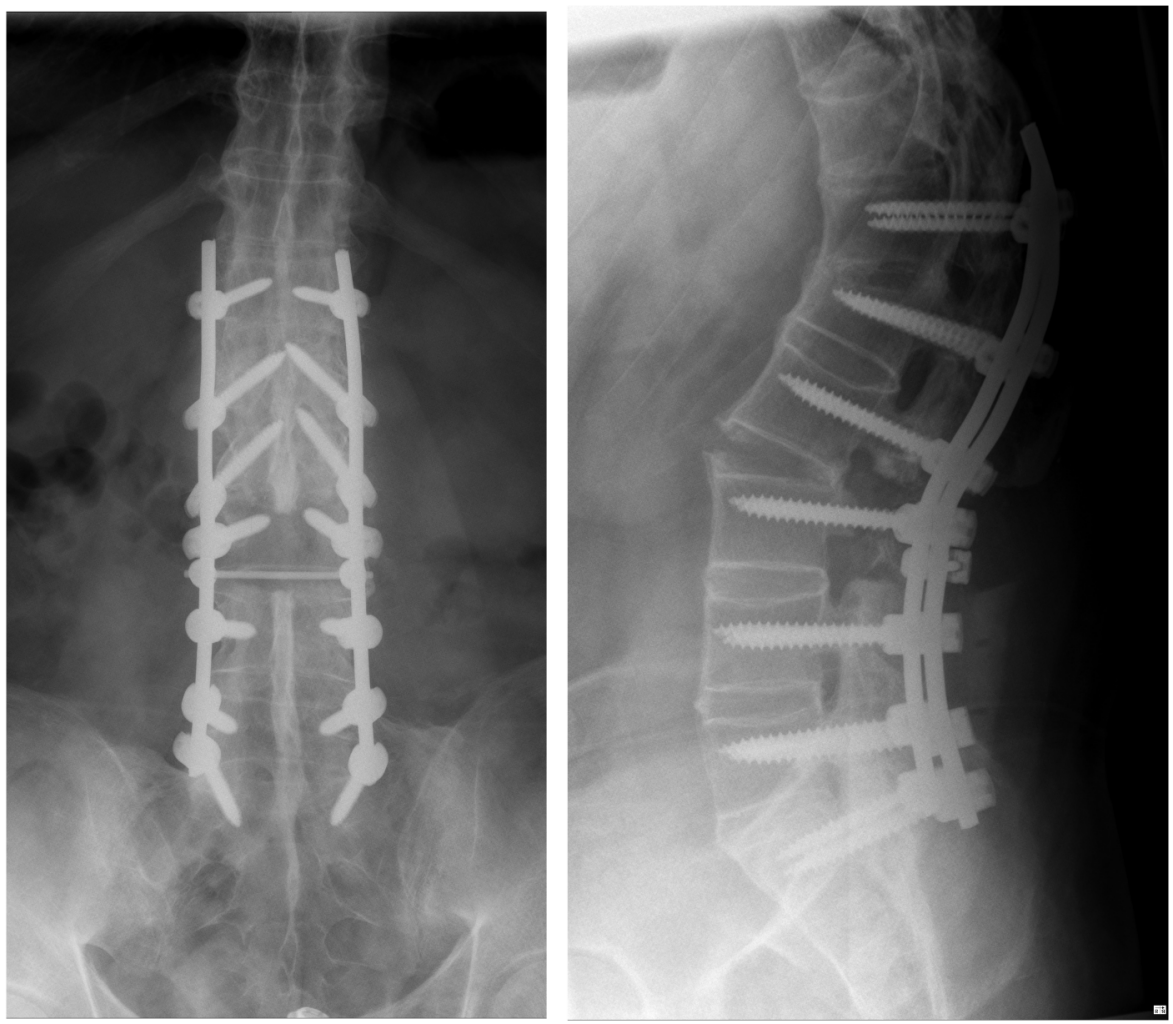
The conventional X-rays of the lumbar spine after the first operation in two planes (ap view left, lateral view right) show the improved position of the spine, however, compared to the intraoperative fluoroscopy (Figure 3), a partial loss of correction.
